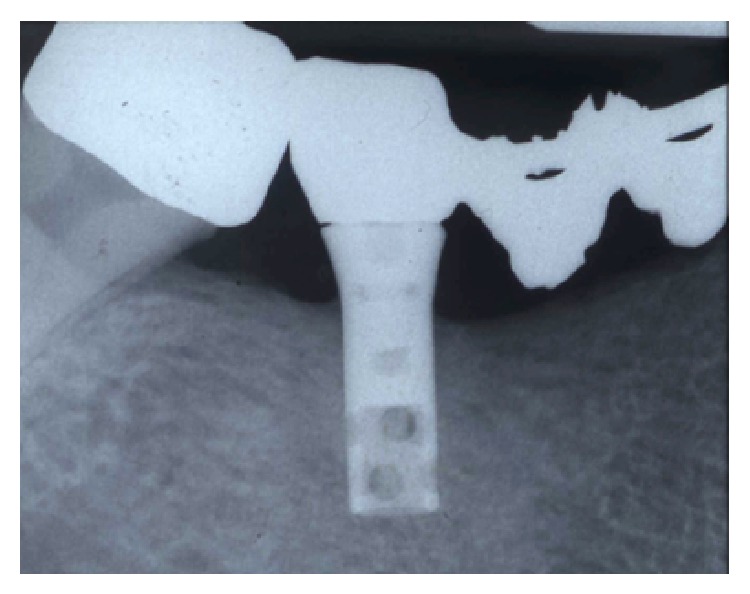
17-year follow-up intraoral radiography. The peri-implant marginal bone levels appeared radiographically stable, without any sign of bone resorption mesially and distally to the implant.
